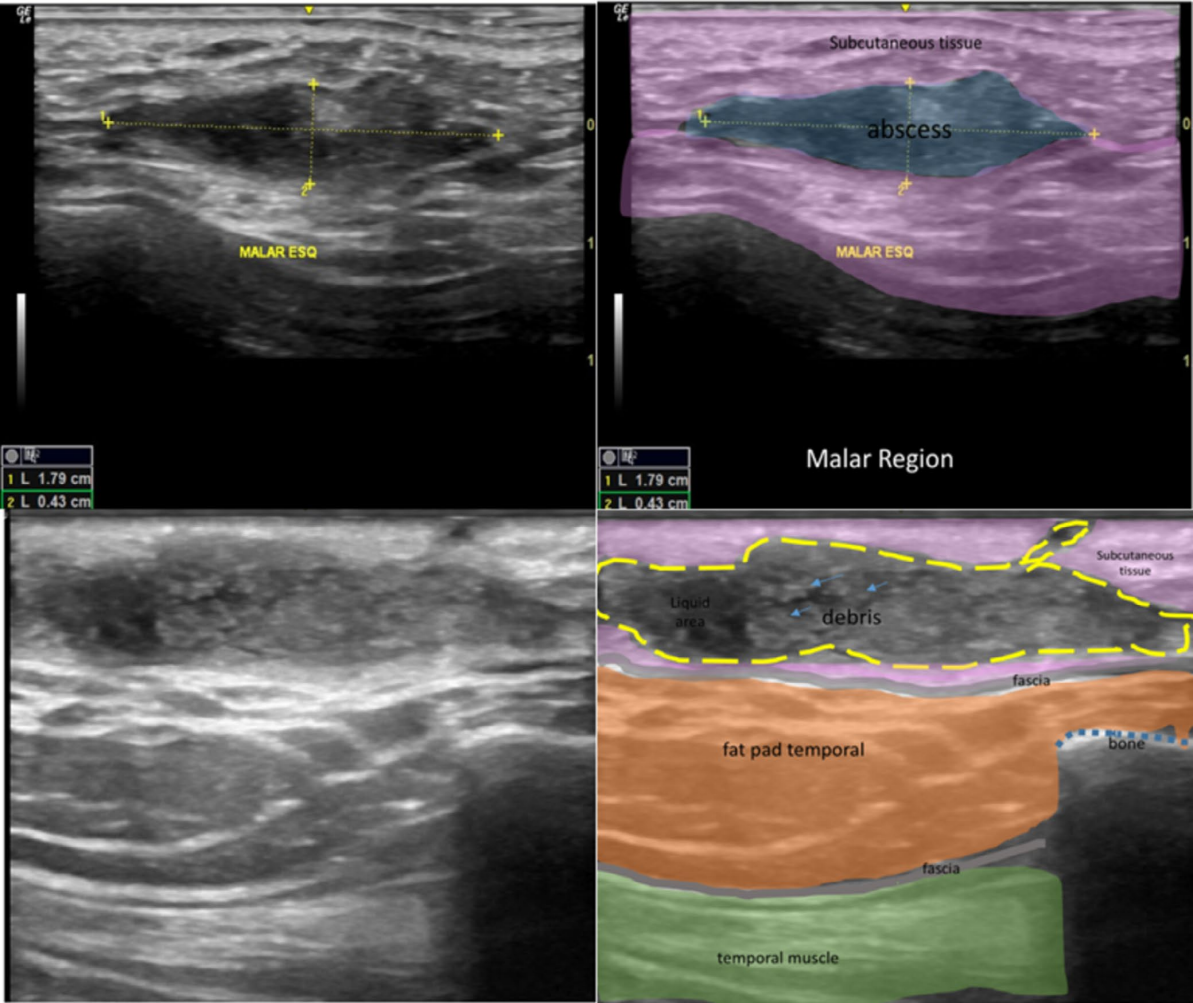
USG images demonstrating two thick fluid collections (abscesses) in the malar and temporal regions. Comparative view: Ultrasound image on the left and annotated image on the right.
